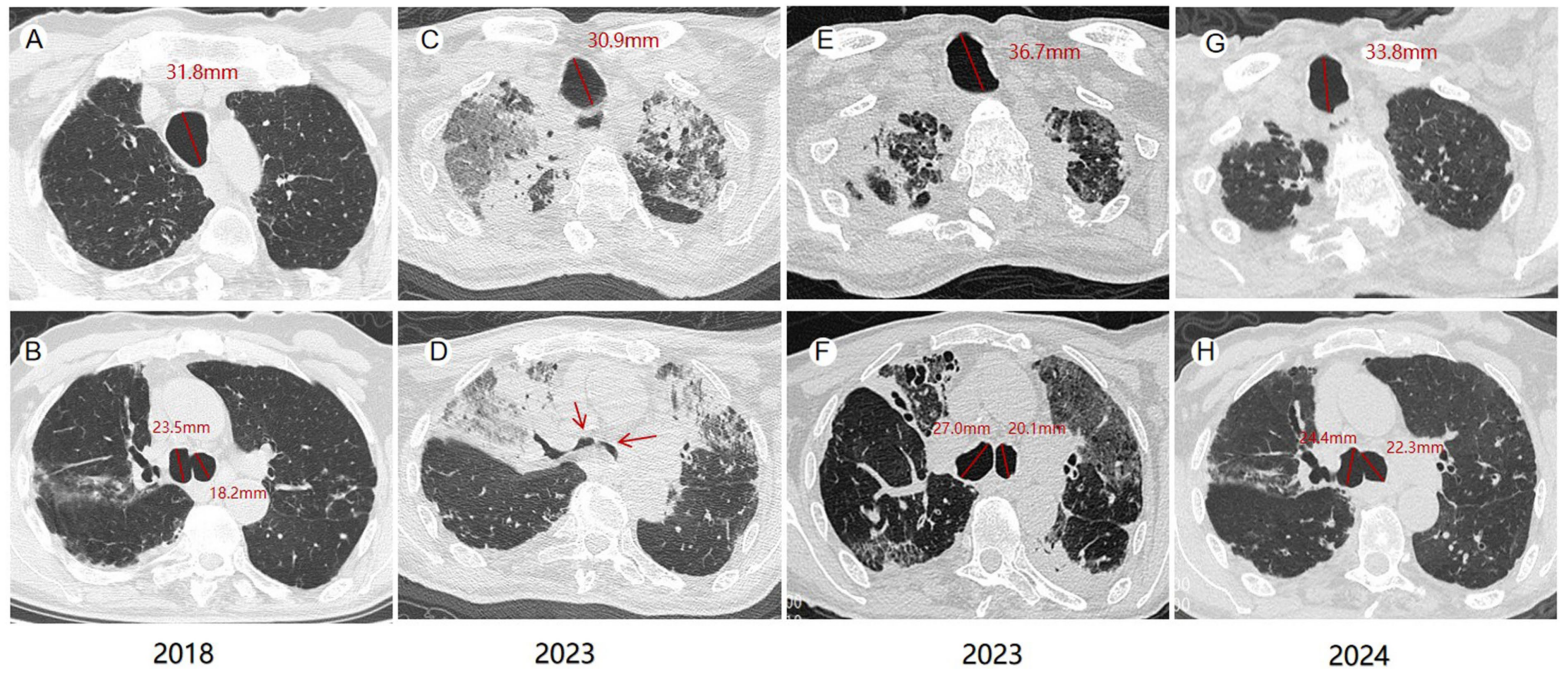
Computed tomography (CT) changes of the patient. In 2018, the maximum diameter of the patient’s trachea, right and left main bronchi were 31.8 mm, 23.5 mm and 18.2 mm (A,B), respectively. Collapse of the tracheal walls during exhalation was seen on CT at admission in 2023 (C,D). The maximum diameters of the trachea, right and left, main bronchi in 2023 were 36.7 mm, 27.0 mm and 20.1 mm, respectively (E,F). Chest CT in 2024 showed that the maximum diameters of trachea, right and left main bronchi were 33.8 mm, 24.4 mm and 22.3 mm, respectively (G,H).
